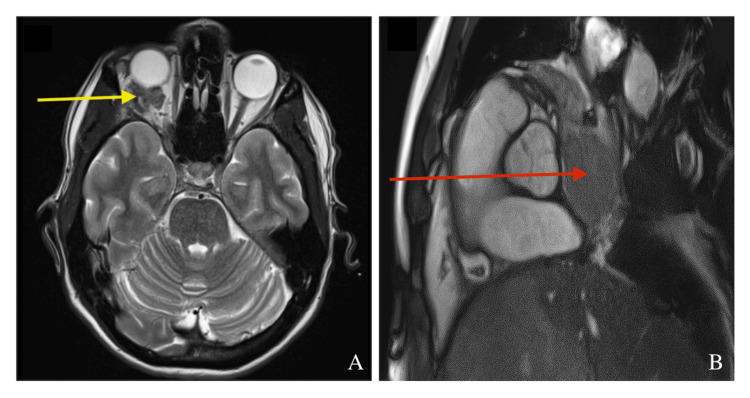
(A) Axial T2-weighted MRI of the brain demonstrating metastatic involvement of the right orbit (yellow arrow), consistent with orbital metastasis from melanoma. (B) MRI of the heart showing a large, non-mobile soft tissue mass (red arrow) obstructing the right main pulmonary artery and involving the right upper and lower pulmonary veins, with extension into and invasion of the left atrium.
